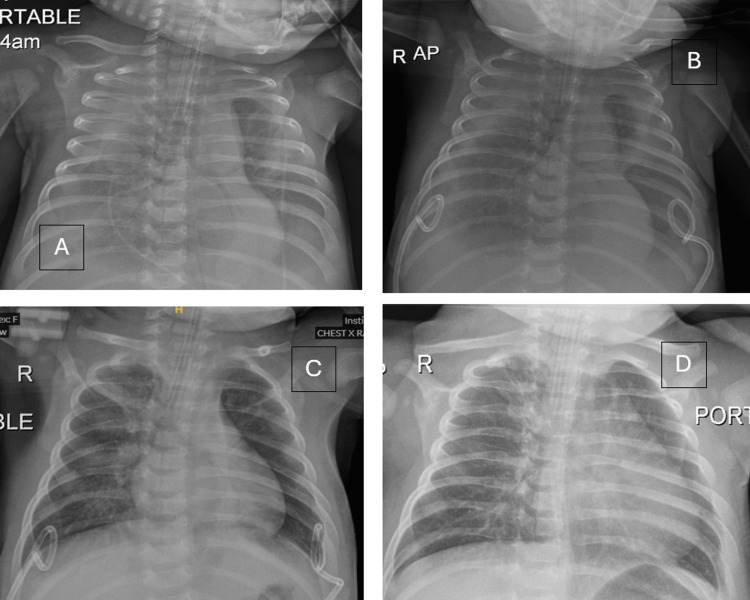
A: Chest radiograph demonstrating marked bilateral pleural effusions with blunting of both costophrenic angles and diffuse lung opacities, worse on the right. B: After insertion of bilateral pigtail catheters, showing early regression of pleural fluid but persistent reduced lung volumes and underlying collapse/consolidation. C: Before removal of pigtails, showing good lung inflation with clear costophrenic angles, indicating near‑complete resolution of effusions. D: After removal of pigtails, demonstrating maintained clear costophrenic angles bilaterally and improved aeration.
